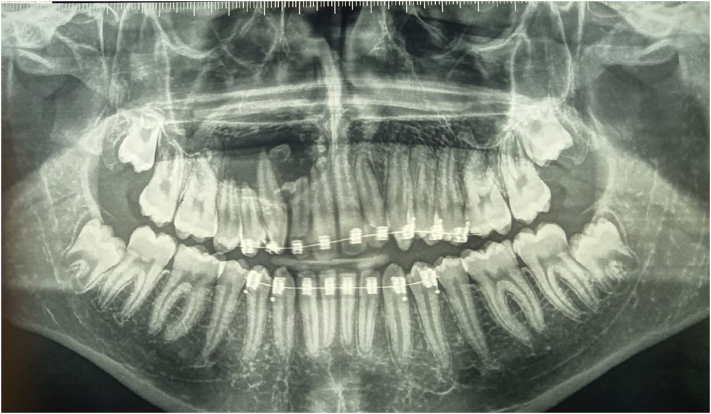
Panoramic radiograph showing a well-defined mixed radiolucent/radiopaque image in the maxillary anterolateral area.
